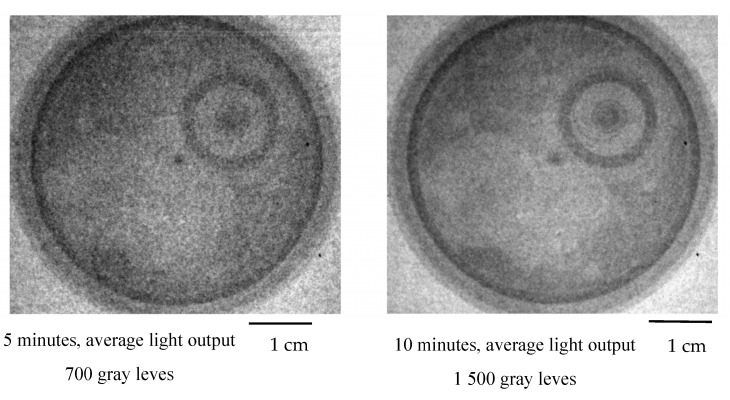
Neutron radiograph of a pocket watch at different exposure times (L/D = 50). Grayscales are stretched for optimal display, and are not the same as Figure 6.
